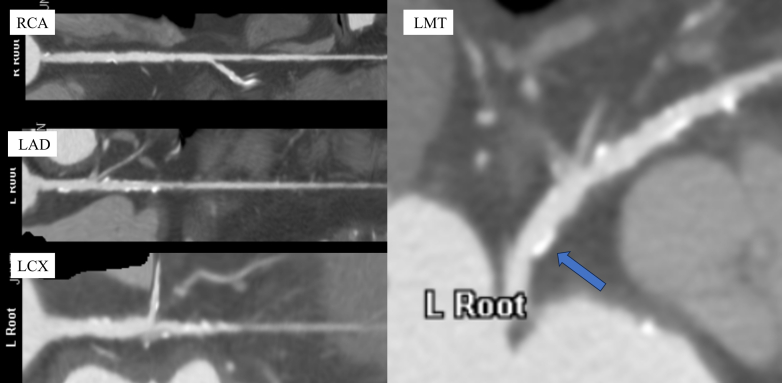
Preprocedural Coronary Computed Tomography Angiography Mild calcification is noted in the left main trunk (arrow), with no stenosis observed. LAD = left anterior descending artery; LCX = left circumflex artery; LMT = left main trunk; RCA = right coronary artery.
